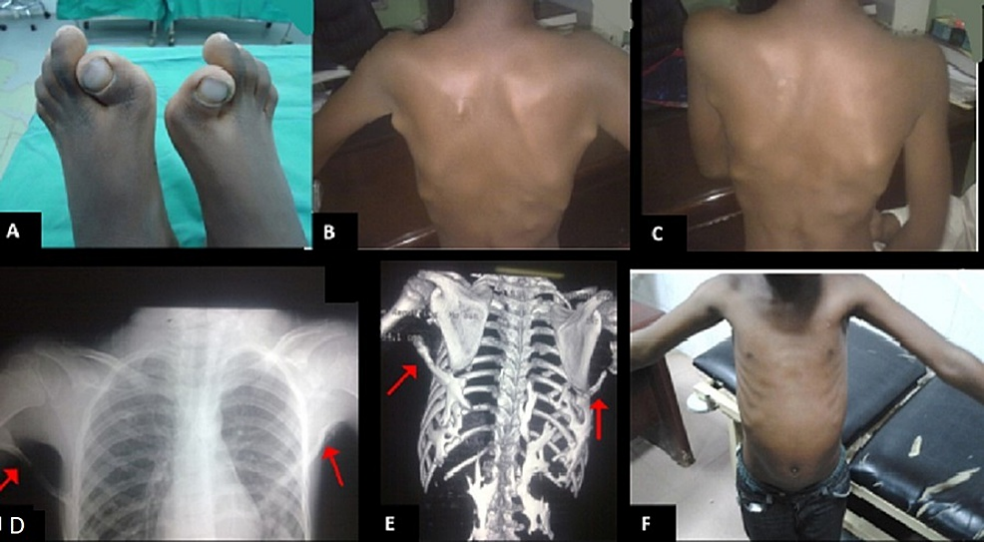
A 10 year boy with bilateral shoulder and back heterotopic ossification. (A) Big toe deformities; limited shoulder (B) abduction and (C) adduction; (D,E) chest X-ray and spine CT scan shows bony bridge (red arrows); (F) 4 months post-operative with gradual loss of shoulder range of movement.
